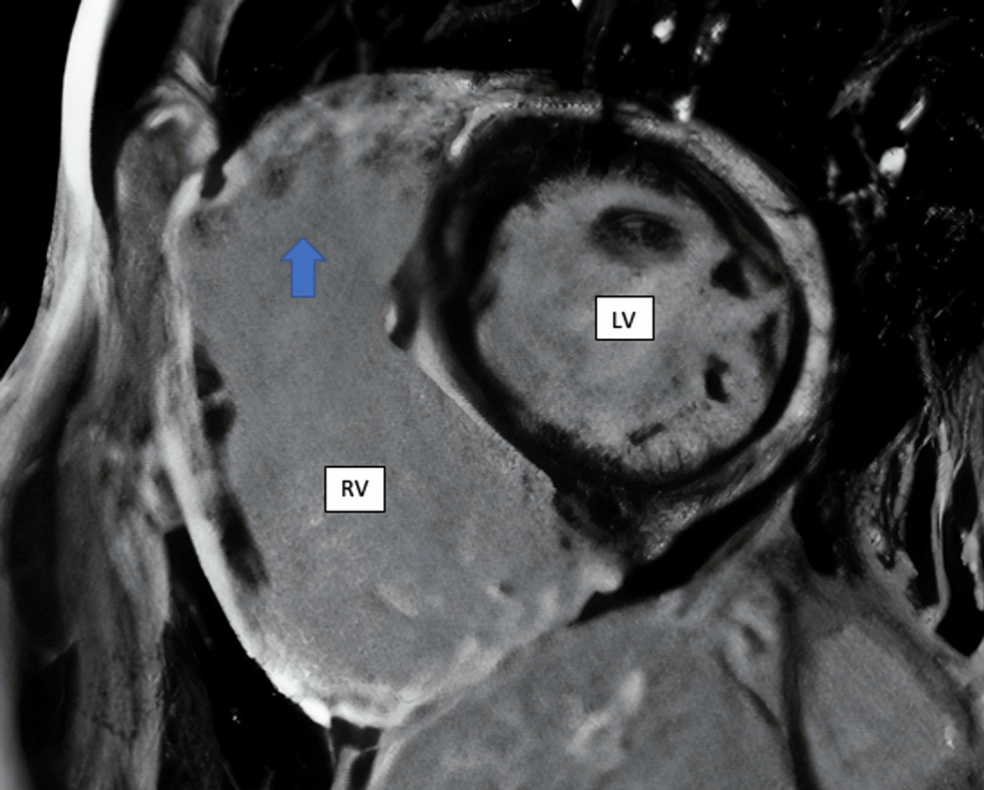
Cardiac MRI featuring dilatation of infundibulum of right ventricle (arrow). RV: right ventricle; LV: left ventricle
